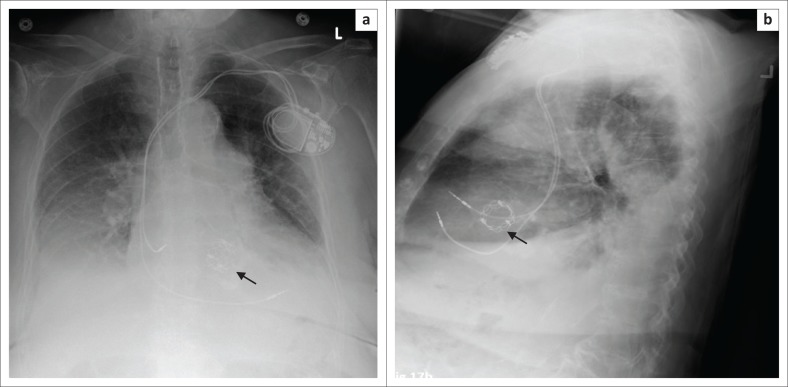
Frontal (a) and lateral (b) chest radiographs showing transcatheter aortic valve replacement (arrow) in an 81-year-old female patient with severe aortic stenosis.
